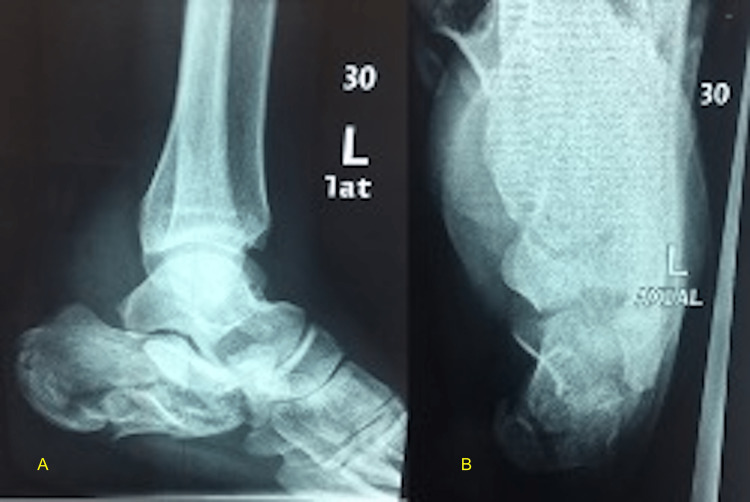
Preoperative radiographs Preoperative radiographs of calcaneus fracture of a 31-year-old gentleman that was operated using the distracted prone positioning. A) Lateral view; B) axial view
